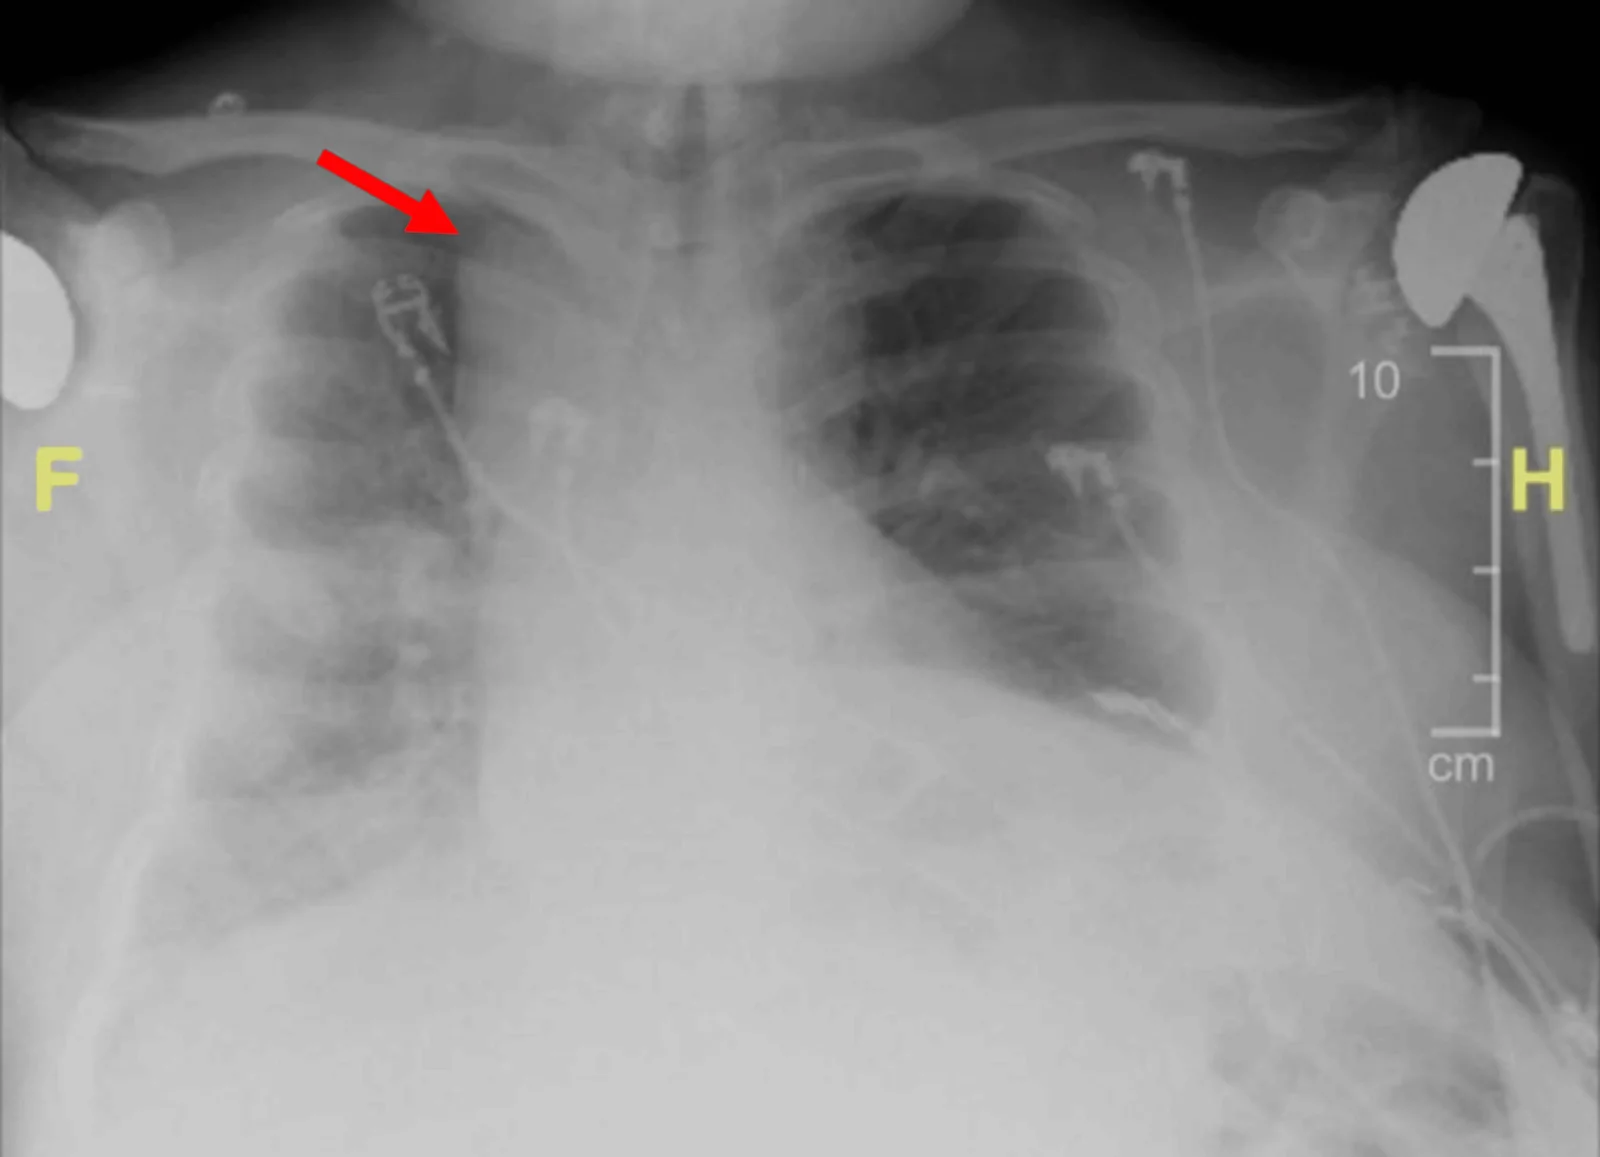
Chest X-ray The chest X-ray demonstrates a right-sided aortic knob, an important radiographic finding that may be misinterpreted as mediastinal pathology. F: field; H: hila
